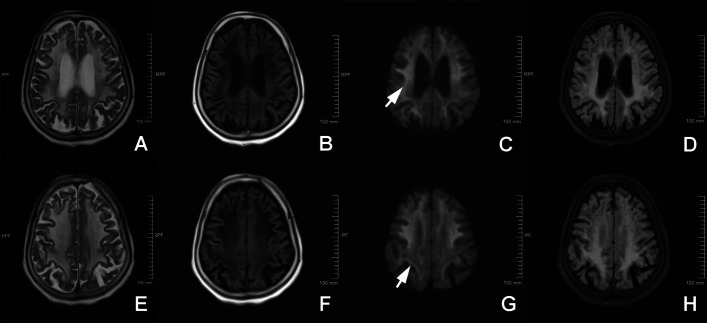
Brain MRI findings. Brain MRI performed on a 1.5-T Siemens scanner. A T1-weighted imaging shows patchy hypointensity. B-C T2-weighted and FLAIR images demonstrate diffuse, symmetric hyperintensities in the periventricular and deep white matter (leukoaraiosis, Fazekas grade 3), including the brainstem and centrum semiovale. D Diffusion-weighted imaging (DWI) discloses a symmetric curvilinear high-intensity signal along the corticomedullary junction (CMJ sign), a characteristic finding of NIID
